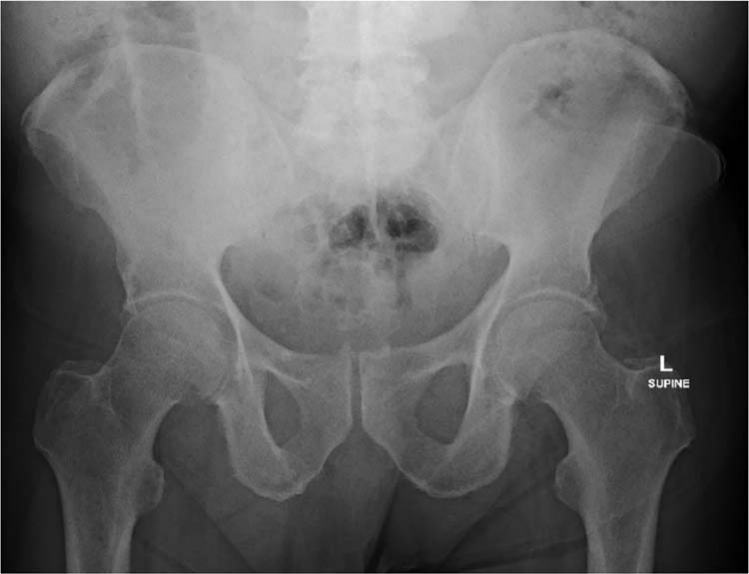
Pelvic X-ray demonstrating passage of second hearing aid.
